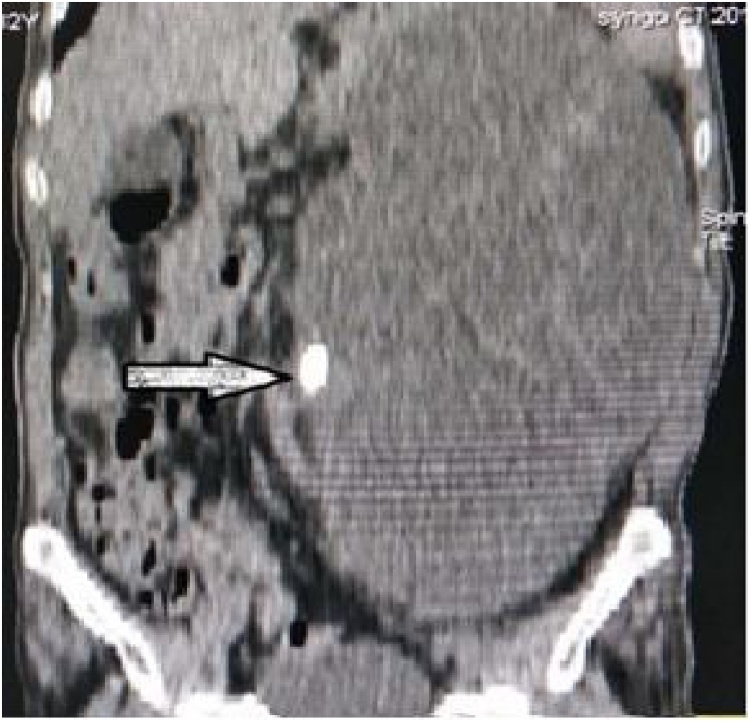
CT scan of the abdomen (coronal section) showing GH, and a high-density shadow (white arrow) located in the renal hilum region.
